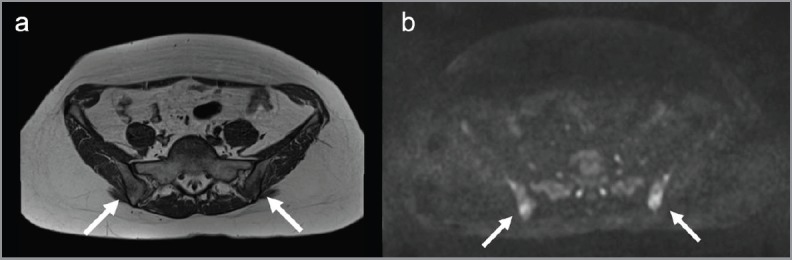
Follow-up MRI. (a). MRI of the pelvis showed the lesions (arrows) not significantly increased in dimensions on T1-weighted images and (b). diffusion-weighted images with b-value of 900 mm/s^2^.
